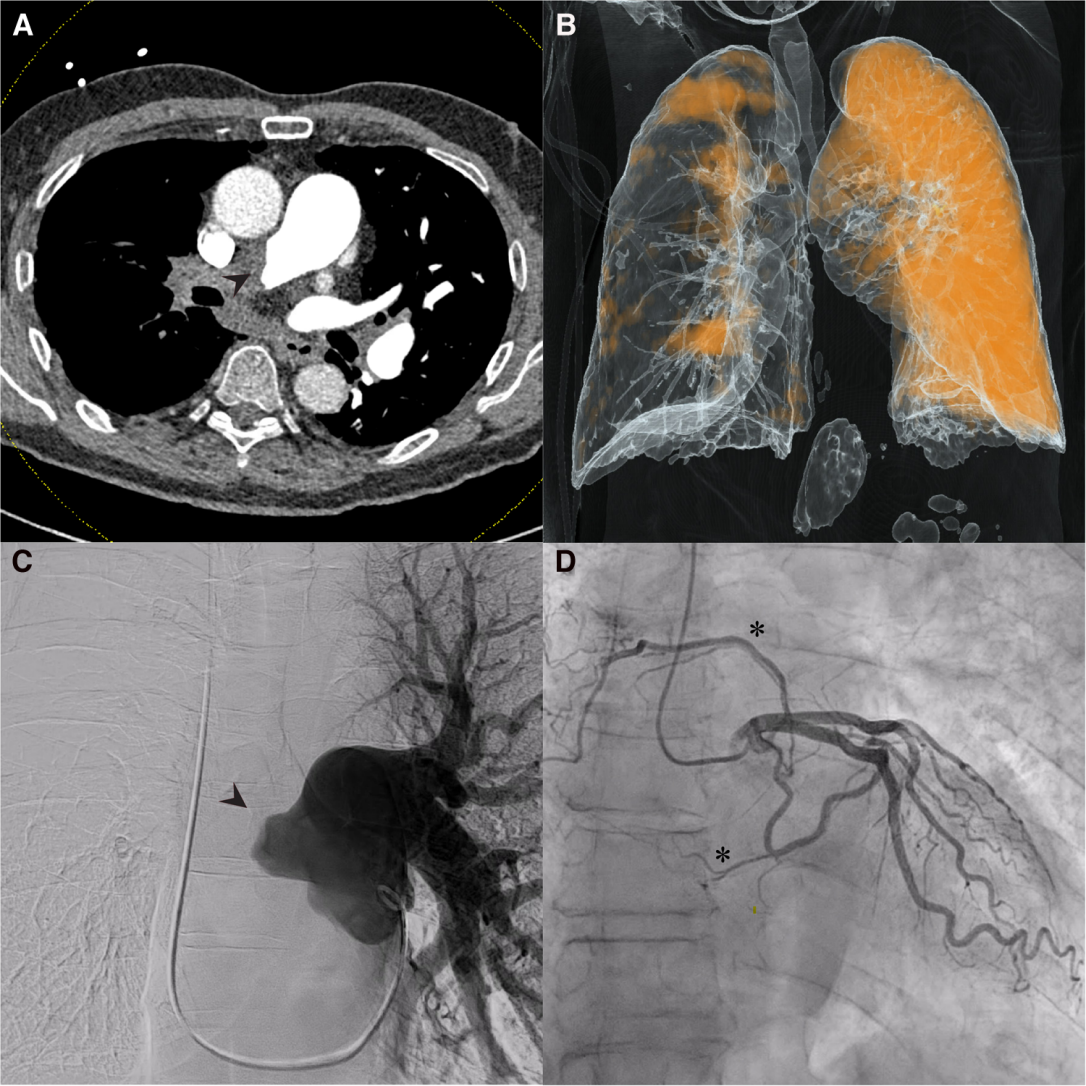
Dual-Energy computed tomography (DECT), pulmonary and coronary angiography. (A) Coronal DECT image demonstrating obstruction of right pulmonary artery (arrowhead); (B) Perfusion mapping demonstrates limited perfusion of right lung from unknown systemic supply at time of imaging. (C) Pulmonary angiography confirmed complete obstruction of right pulmonary circulation (arrowhead); (D) Coronary angiography revealed multiple systemic coronary-pulmonary collaterals from the left circumflex artery (asterisks).
